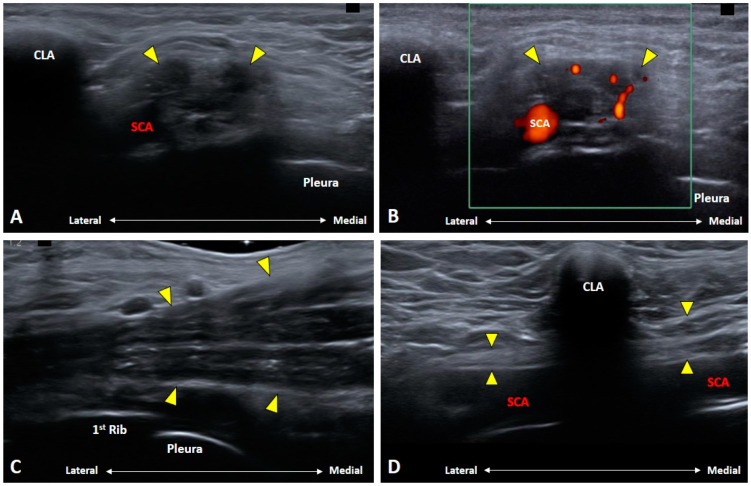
Ultrasound imaging of metastatic brachial plexopathy. The nerves (yellow arrowheads) are enlarged (A) with intra-neural hypervascularity (B). In the long-axis view, segmental swelling of the nerves (yellow arrowheads) are seen at the supraclavicular (C) and infraclavicular (D) levels. CLA: clavicle; SCA: subclavian artery.
